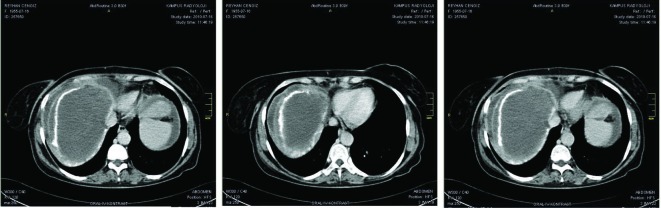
An abdominal CT scan demonstrated multiple hepatic masses in the right lobe of the liver, the largest of which was 12 cm in diameter, non-uniform contrast enhancement of the liver parenchyma, an enlarged caudate lobe, and hepatic and portal vein thrombosis with ascites.
